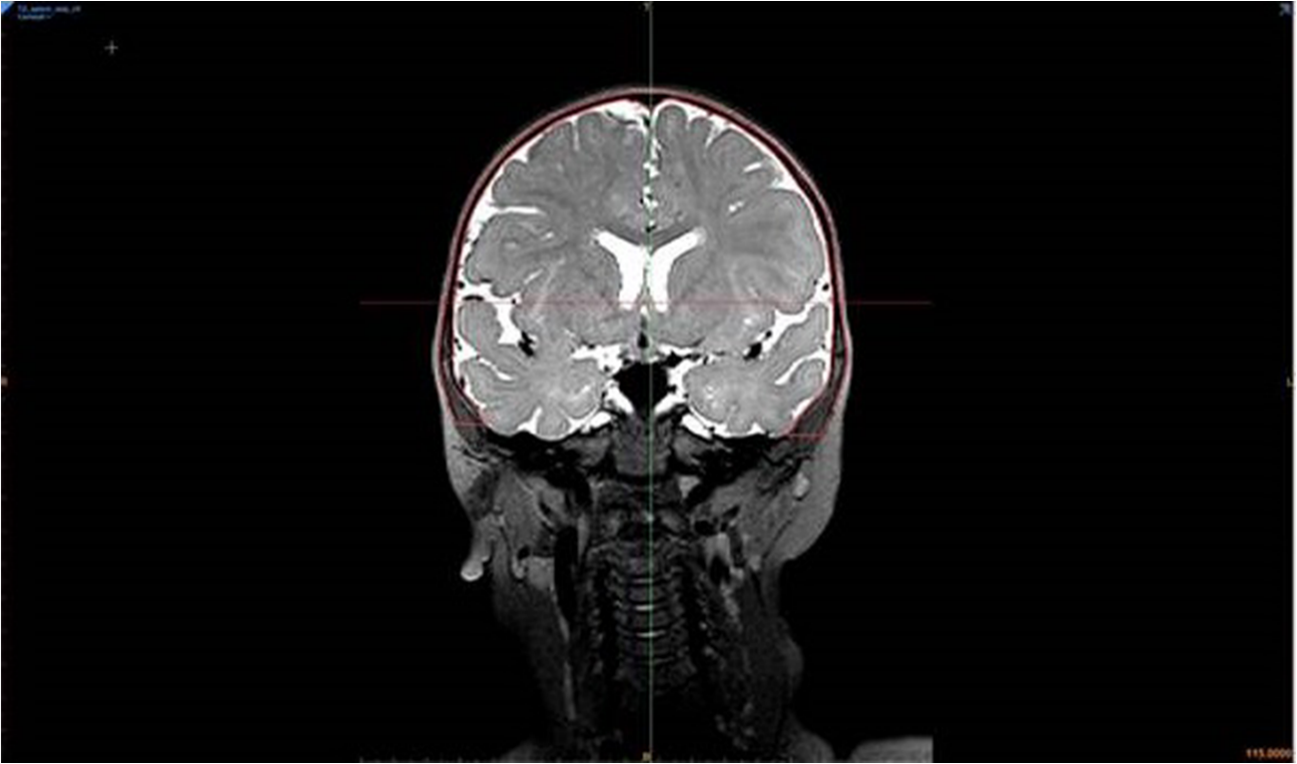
Screenshot of a MRI in coronal plane in a 1-year-old girl with trigonocephaly. The skull bone is segmented and traced with red lines
